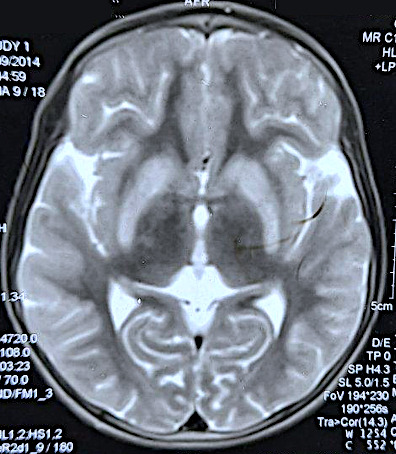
MRI (T2WI) of the brain showing abnormal hyperintensity in bilateral basal ganglia.
